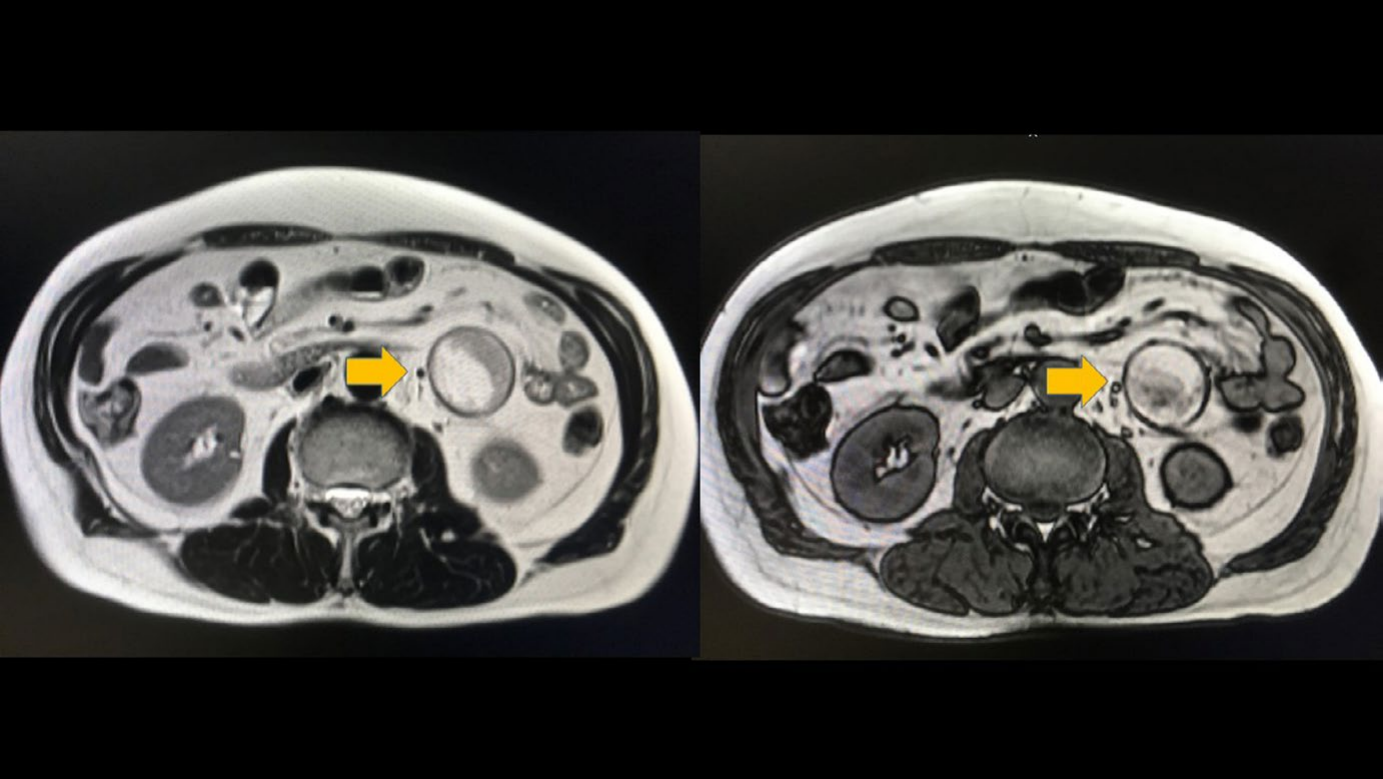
Left: Magnetic resonance imaging T1W and Right: Magnetic resonance imaging T2W showed a round lesion with a thick hypointense wall and a central fatty focus
